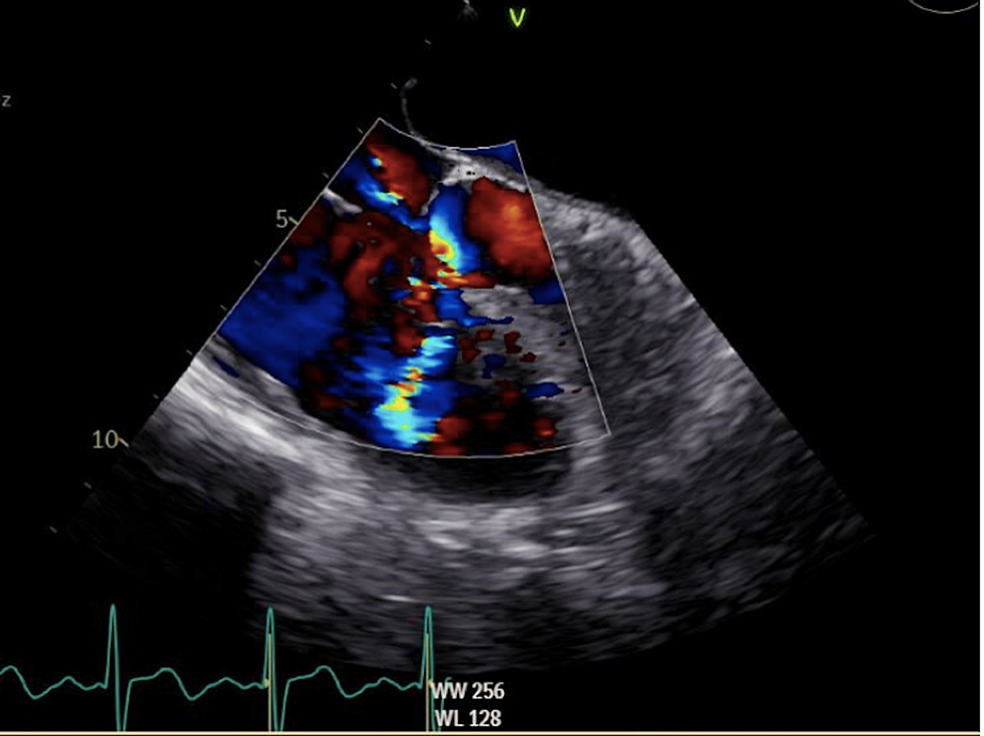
TEE image with color Doppler flow through the VSD. TEE, transesophageal echocardiogram; VSD, ventricular septal defect
